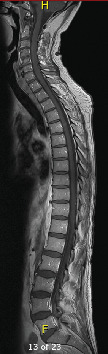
MRI of the entire spine showing no evidence of leptomeningeal carcinomatosis. Sagittal T1-weighted postcontrast MRI of the cervical, thoracic, and lumbar spine shows no abnormal leptomeningeal enhancement or evidence of spinal metastases. Findings were radiographically unremarkable despite positive cerebrospinal fluid cytology, underscoring the diagnostic limitations of imaging in leptomeningeal carcinomatosis (original image, Department of Medical Imaging, Western University).
